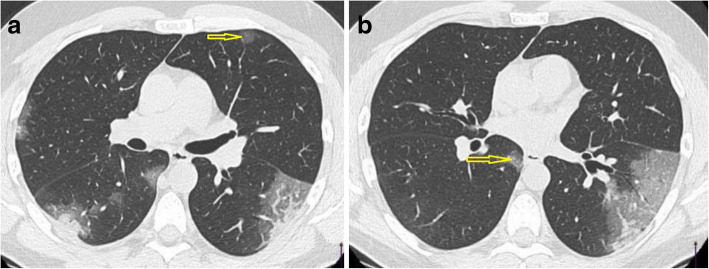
CT chest axial cuts of COVID-19 patient. A: ground glass opacity (anterior segment of left upper lobe, yellow arrow) and combination of GGO and consolidation in both lower lobe (peripheral, subpleural). B: ground glass opacity (medial segment of right lower lobe, yellow arrow) as well as GGO and consolidation of left lower lobe (peripheral, subpleural)
